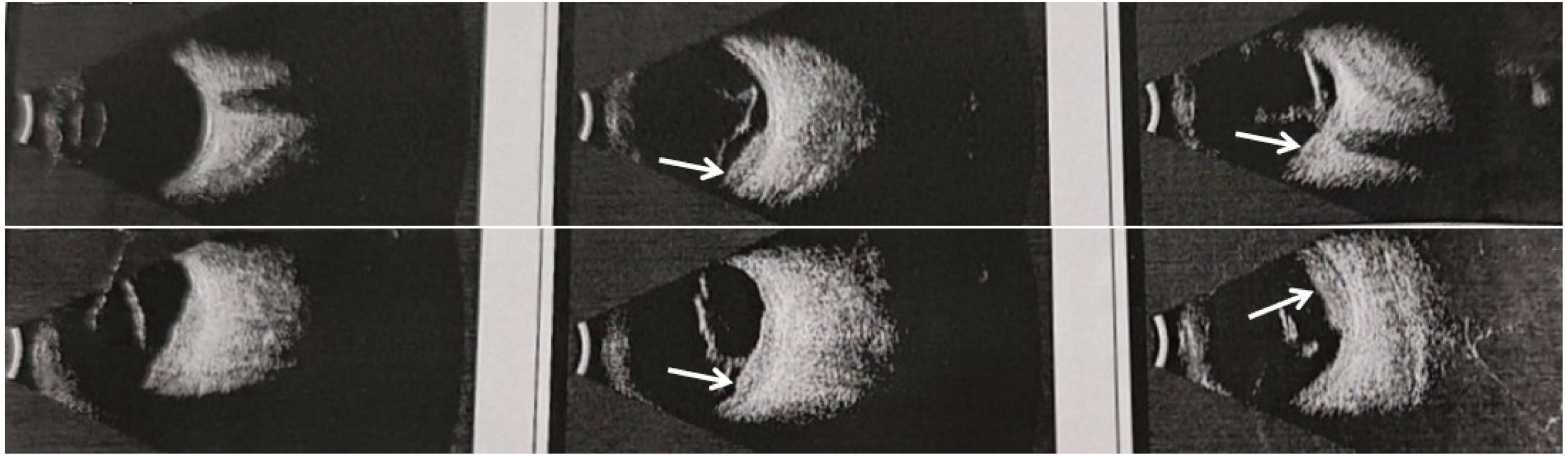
Ultrasonography of the left eyeball showing thickening of the choroid at the posterior pole, suggestive of an intraocular space-occupying lesion.
